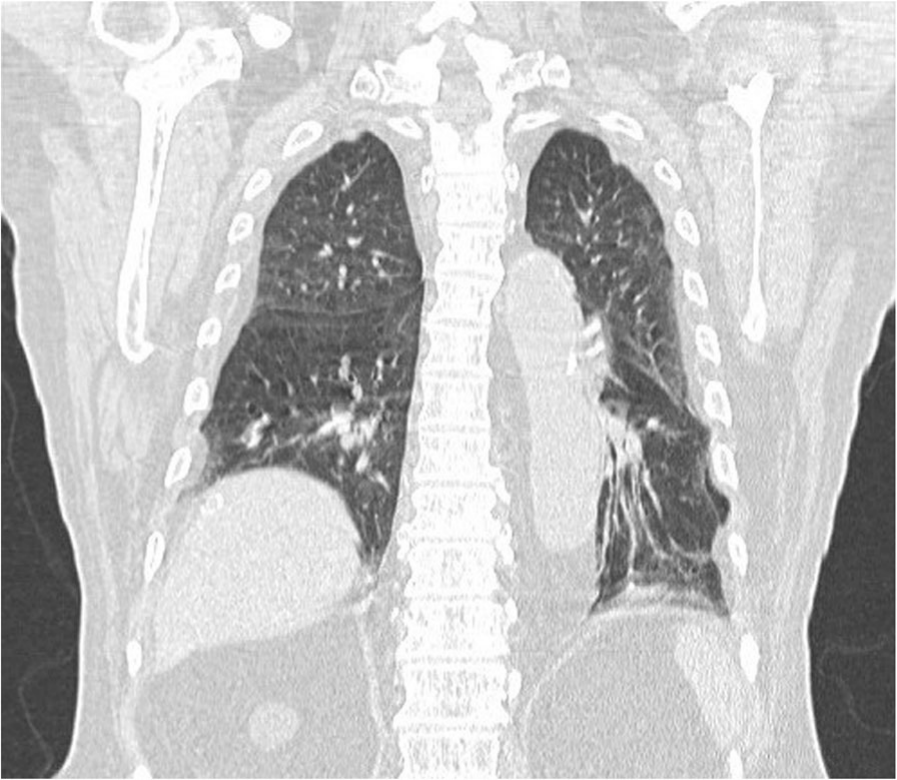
A computed tomography scan from a year prior to presentation shows a normal aorta
